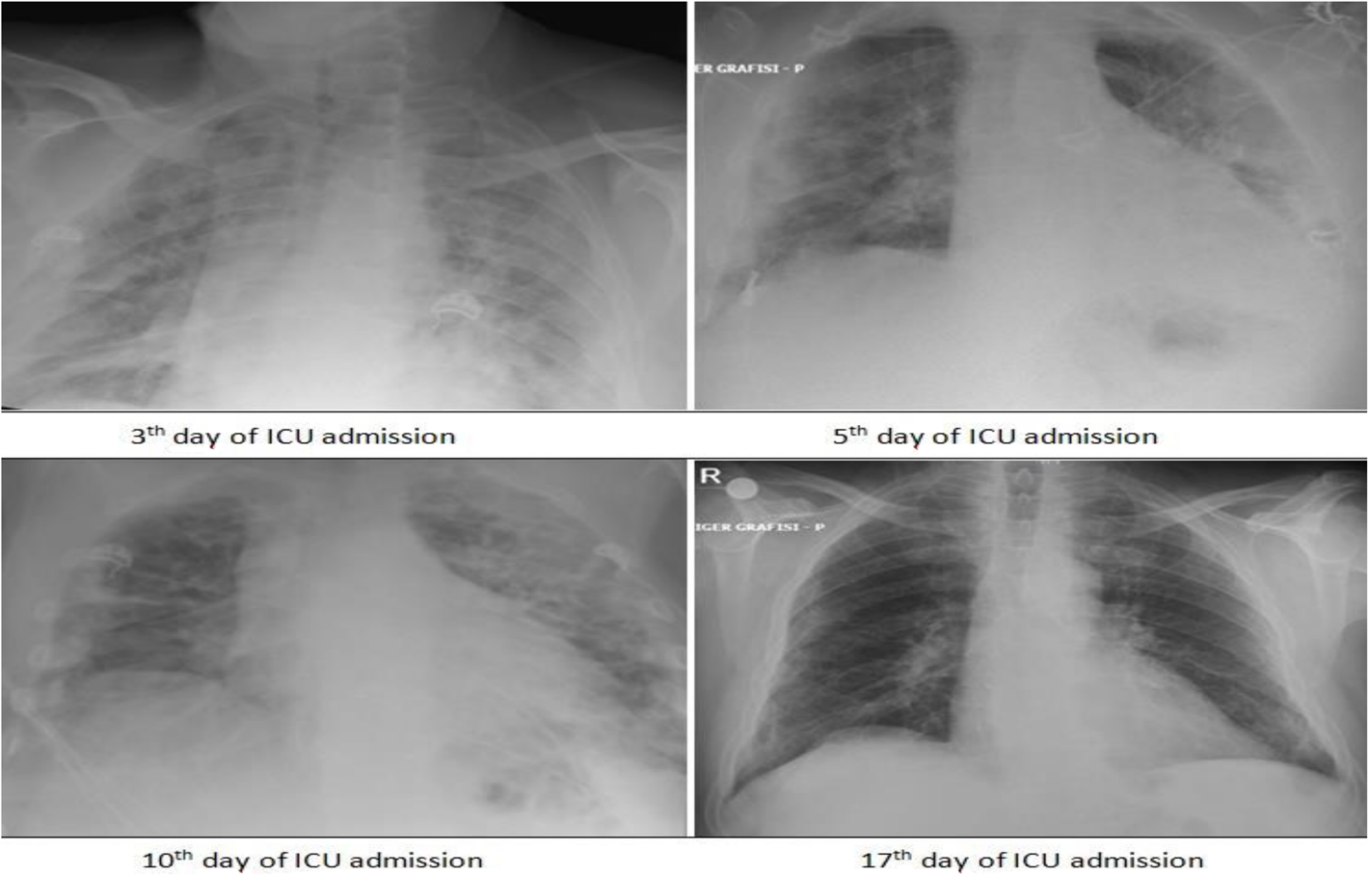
Intermittent PA chest radiographs during ICU stay
